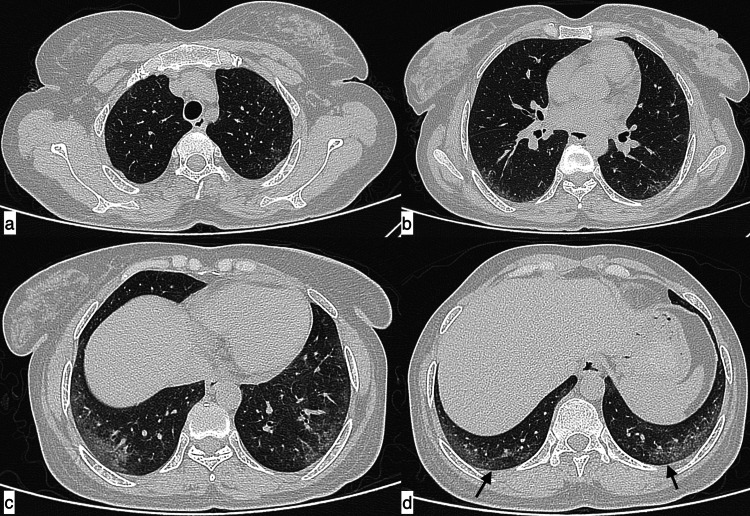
NSIP pattern of ILD A 42-year-old female patient with SLE presented with a four-month history of mild cough and shortness of breath. Axial HRCT chest images in the lung window (a-d) revealed ground-glass opacities with interlobular interstitial septal thickening located peripherally in both lung fields with an apicobasal gradient and subpleural sparing (black arrows), suggestive of the NSIP pattern of ILD. SLE: systemic lupus erythematosus; HRCT: high-resolution CT; NSIP: nonspecific interstitial pneumonia; ILD: interstitial lung disease
